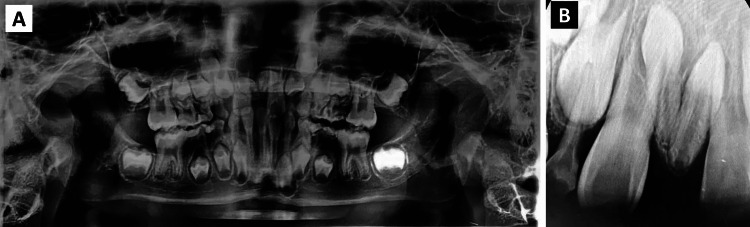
Preoperative radiographs revealing bilateral impacted and inverted mesiodentes. (A) Panoramic radiograph. (B) Periapical radiograph
